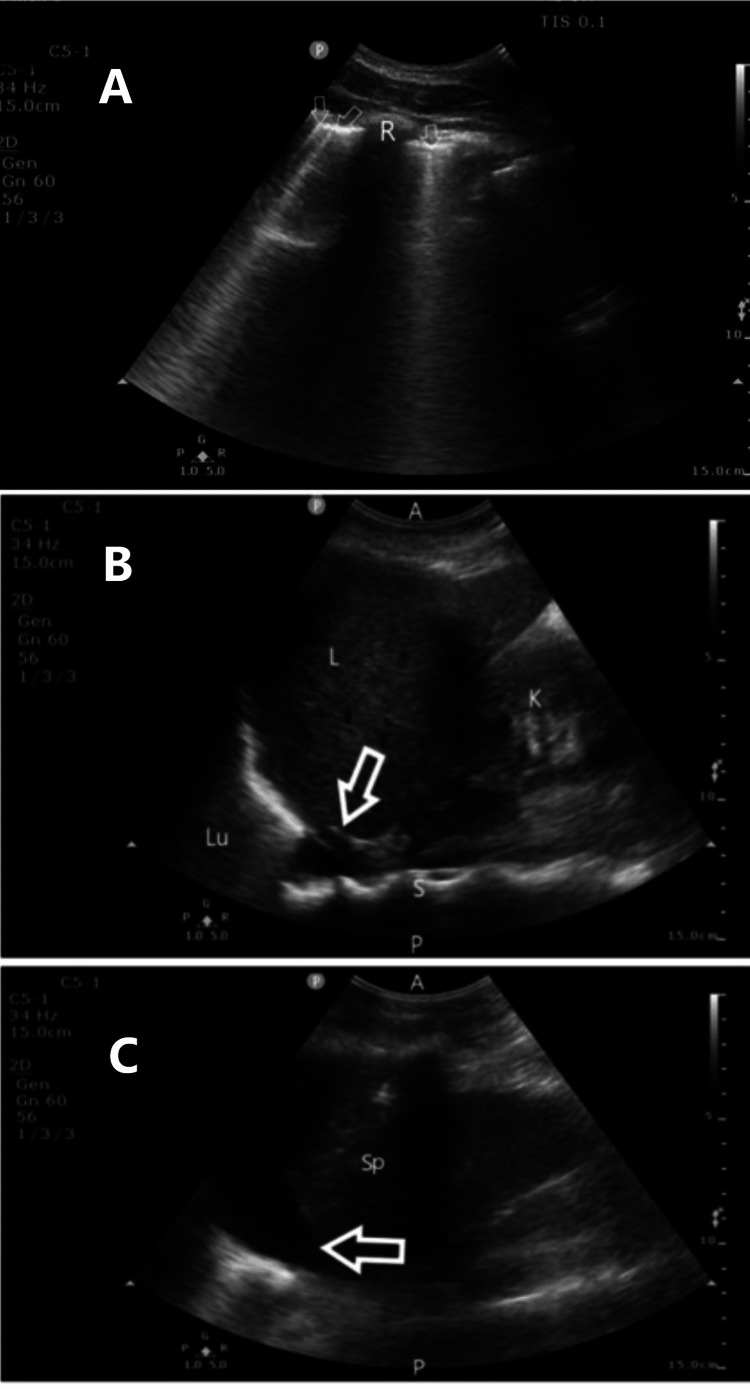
Pulmonary edema and pleural effusions Image A: Upper thorax lung field. Multiple B-lines (arrows) throughout lung fields indicating pulmonary edema. R, rib. Image B: Right-upper-quadrant ultrasound. Arrow indicates pleural effusion and clearly visible "spine sign" where thoracic spine is visible behind fluid collection. A, anterior, P, posterior, Lu, lung, S, spine, K, kidney, L, liver. Image C: Left-upper-quadrant ultrasound. Arrow indicates pleural effusion. Sp, spleen.
